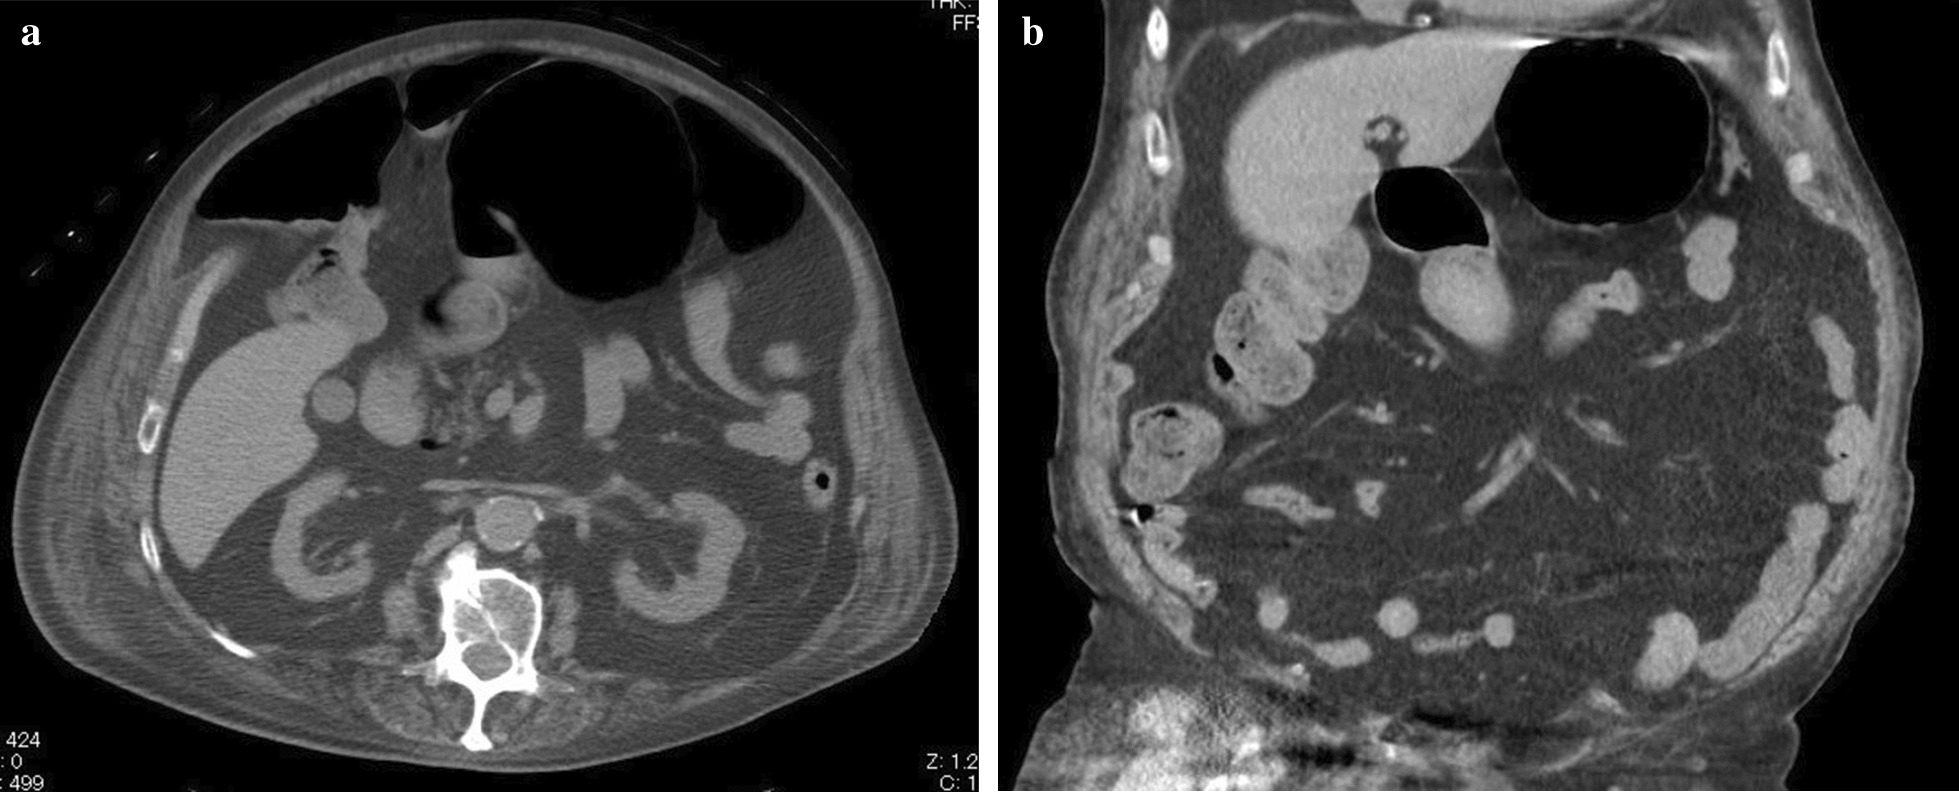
Plain abdominal CT revealing distended stomach and thinning of the left abdominal wall muscles. a Axial view; b Coronal view
